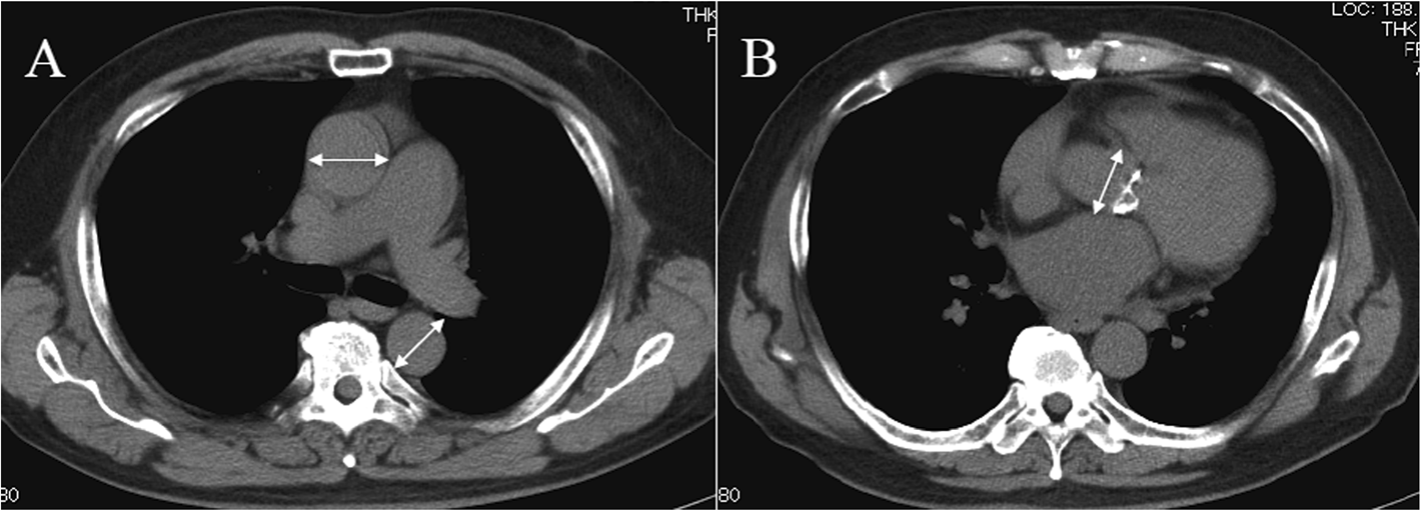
Measurements of the aortic dimension. A. The measurements of the diameter of the ascending aorta (AAo) were obtained from the largest diameter in the transverse plane of preoperative CT and the diameter of the descending aorta (DAo) was obtained from the same CT slice. B. The measurements of the aortic root (AoR) were obtained from the diameter of the sinus of Valsalva.
